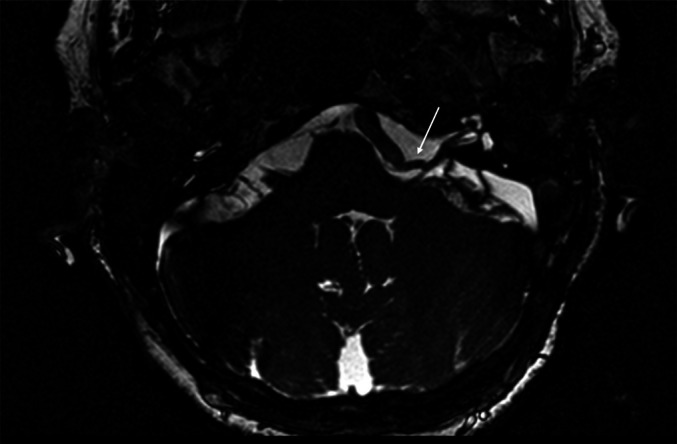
Postoperative MRI of the brain. Resolution of the neurovascular conflict in the left cerebellopontine angle between AICA (white arrow) and facial nerve at its REZ after transposition of the offending vessel
